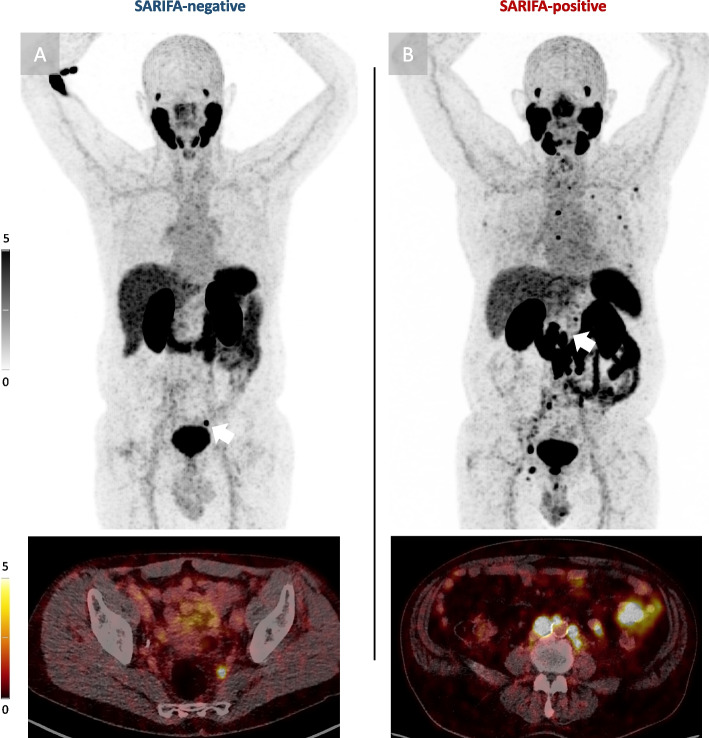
Exemplary maximum intensity projections of PSMA-PET/CT at point of first biochemical recurrence. A SARIFA-negative pT3a prostate cancer patient with a PSMA-positive iliaco-internal lymph node (marked by white arrow). B SARIFA-positive pT3a prostate cancer patient with PSMA-positive regional as well as distant lymph node (marked by white arrow) involvement as well as several bone metastasis. Distant lymph nodes were defined as all extra-pelvic lymph node manifestations. pT: depth of invasion, PET/CT: Positron emission tomography/Computed tomography, PSMA: prostate-specific membrane antigen, SARIFA: Stroma-AReactive-Invasion-Front-Areas SUV: standardized uptake volume
